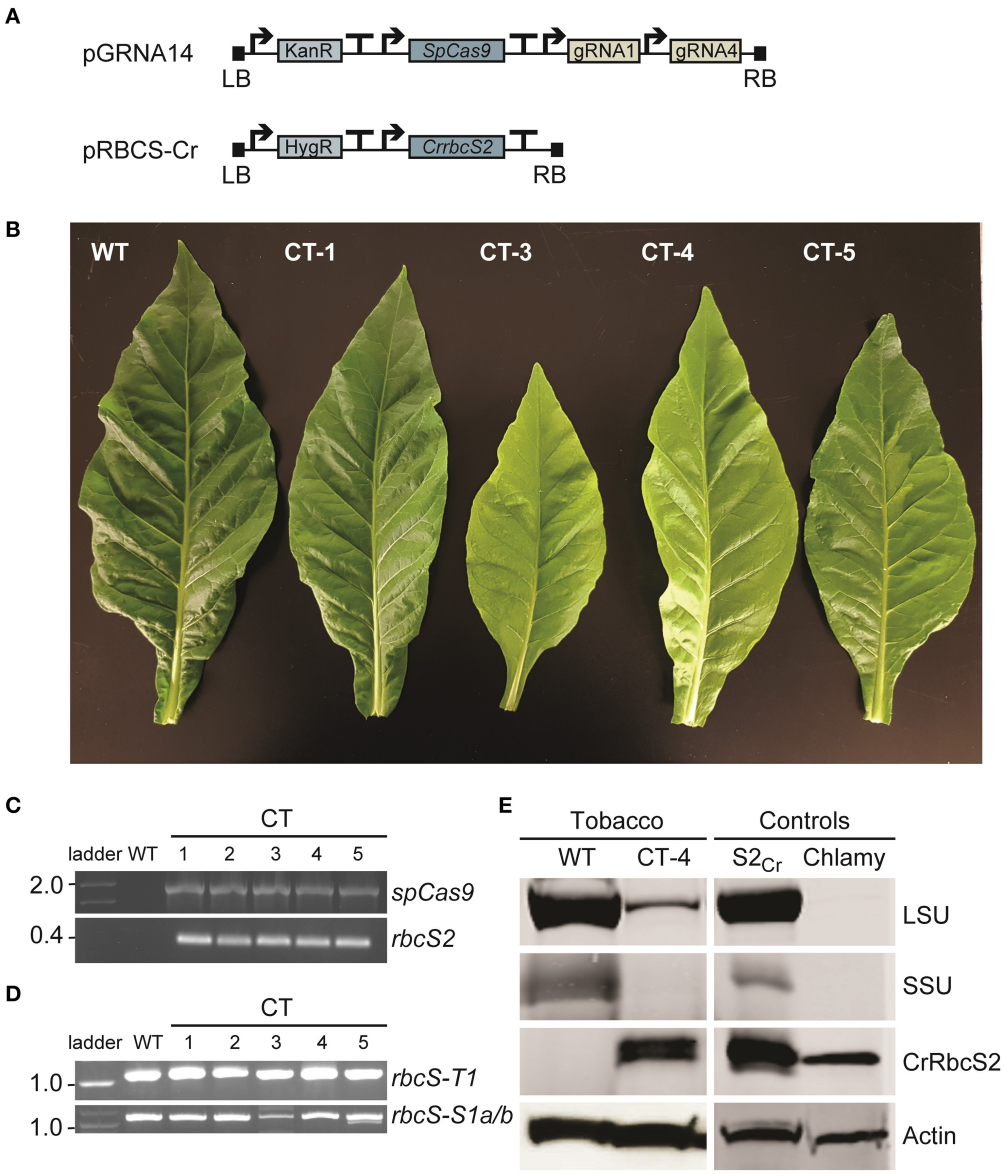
Phenotypes and molecular analyses of co-transformed (CT) plants. (A) Wild-type plants were transformed with a Cas-gRNA vector (vector pGRNA14) and gene expression cassette for *CrrbcS2* from Chlamydomonas (vector pRBCS-Cr) (see Supplementary Figures 1, 2 for vector details). (B) Leaves of a wild-type (WT) plant and four CT lines with phenotypes ranging from wild-type-like (CT-1) to pale (CT-3 and CT-4) and chimeric (CT-5) are shown. (C) PCR amplification of cDNA showing stable integration and expression of *SpCas9* and *rbcS2* in T_0_ CT lines. (D) PCR amplification of *rbcS-T1* and *rbcS-S1a/b* (as in Figure 2B). (E) Immunoblots of total soluble protein to detect the Rubisco large subunit (LSU) and small subunit (SSU) from tobacco and SSU from Chlamydomonas (CrRbcS2) in CT-4 (T_1_ generation). The two controls were from Arabidopsis expressing CrRbcS2 (S2_Cr_ ; Atkinson et al., 2017) and Chlamydomonas. An actin loading control is shown. No LSU and SSU bands were visible for the Chlamydomonas extract using the primary antibody against wheat Rubisco.
